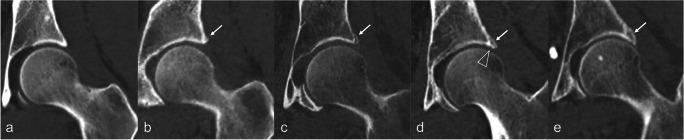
Coronal reformats of CT examinations of five hips showing different sizes of acetabular rim ossifications in the posterosuperior quadrant (arrows). a No ossifications (b–e) acetabular rim ossification with increasing size from left to right. Note the double-rim sign, which has been described for the diagnosis of labral ossification (two lines are visible at the location of the ossification, formed by the contour of the acetabular rim and that of the ossification). Also note a small notch between the acetabulum and the ossified labrum in d, likely corresponding to the chondrolabral recess, which has been described as a diagnostic clue to differentiate labral ossifications from osteophytes [4]
